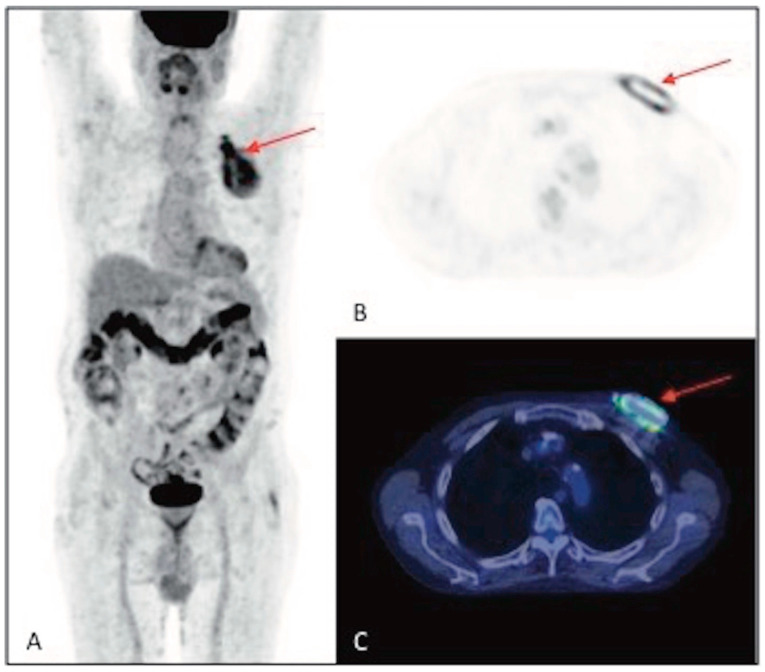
^18^F-FDG PET/CT of a 73-year-old man with implanted ICD, with suspected CIEDI due to the presence of local signs of infection and positive blood culture for *Staphylococcus aureus*. (A) MIP, (B) axial PET and (C) axial fusion images demonstrate the presence of intense and nonhomogeneous increased ^18^F-FDG uptake around the device, at the generator pocket (SUV_max_ 12.9) (red arrows). After the extraction of the device, microbiological analysis confirmed the infection caused by the pathogen *Staphylococcus aureus*.
